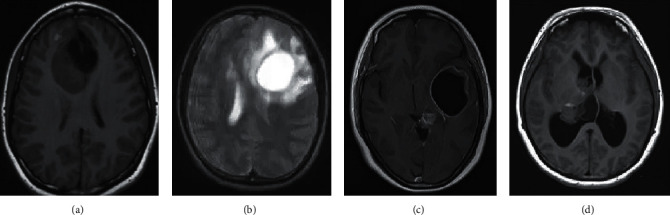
MRI images of patients with glioma in grade IV. The patient was a female, 62 years old. (a, b) MRI plain scan images and (c, d)MRI enhanced images.
